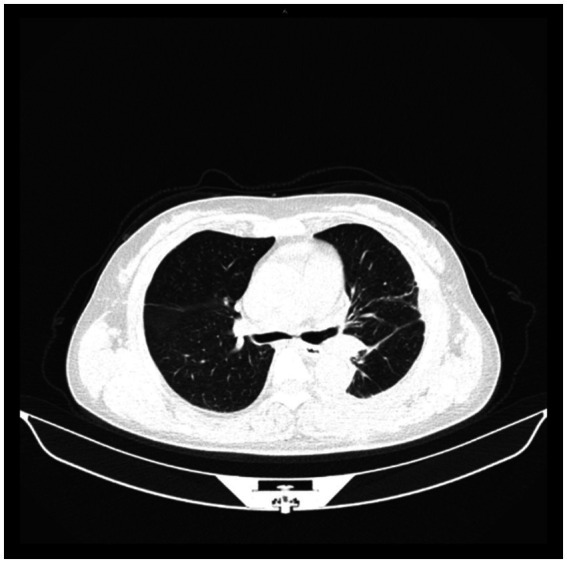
Chest CT at the time of reexamination after discharge from hospital.
